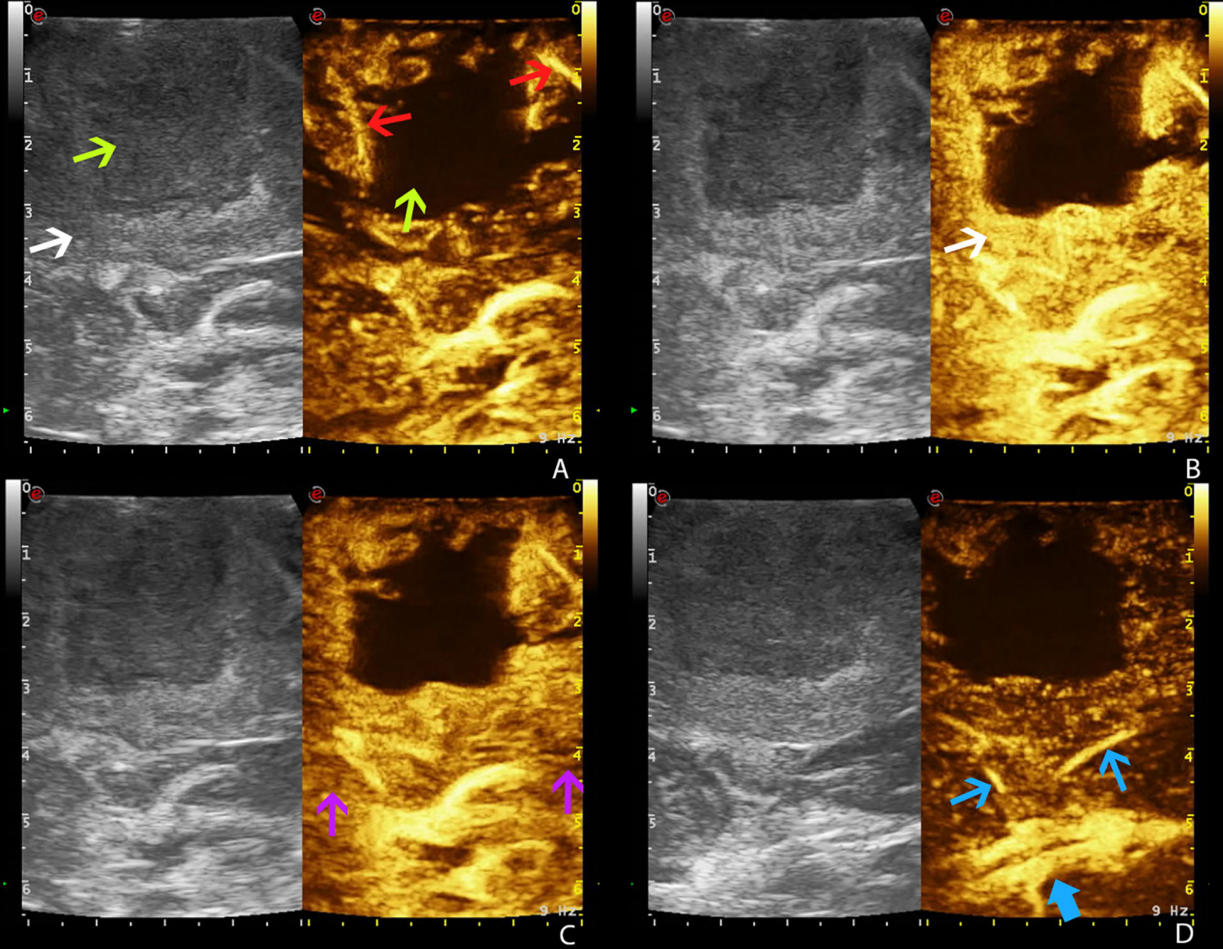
Time frame of GBM visualization by CEUS (case no. 3) (dual frame visualization: B-mode imaging on the left, contrast-enhanced ultrasound imaging on the right). Gas-filled microbubble contrast agent allows to visualize the different phases of intravascular contrast-enhancement thus visualizing the tumor angioarchitecture. (A) Arterial phase. (B) CE peak. (C) Parenchymal phase. (D) Venous phase. White arrow: GBM. Green arrows: necrotic core of GBM. Red arrows: GBM arterial supply. Purple arrows: normal brain parenchyma. Blue arrow: GBM venous drainage (blue thick arrow: internal cerebral veins).
